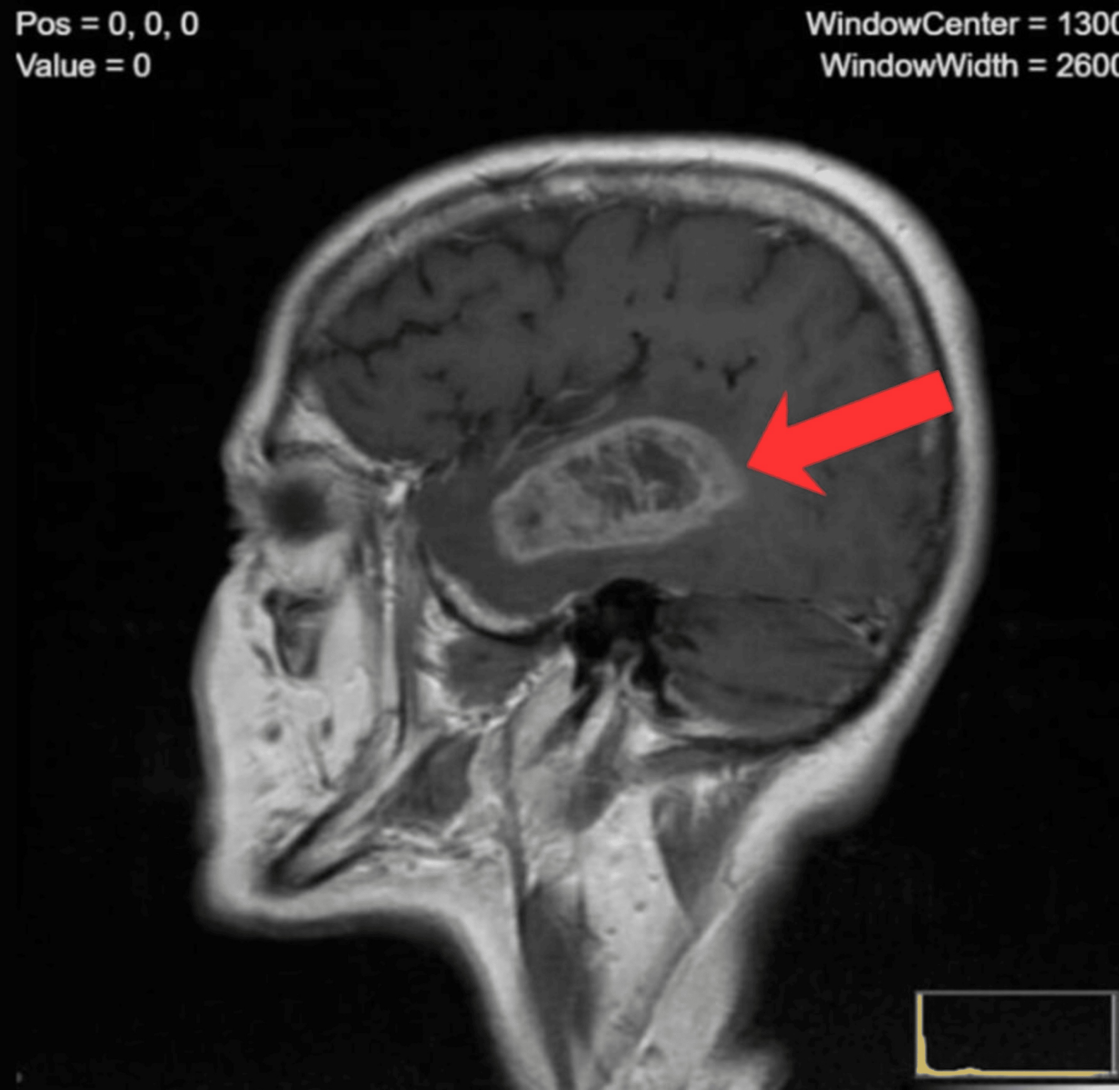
Sagittal contrast-enhanced MRI of a right temporal lobe mass Sagittal contrast-enhanced MRI demonstrating a large heterogeneous mass in the right temporal lobe with irregular ring enhancement and central necrosis. The lesion invades deep cerebral structures and produces a marked mass effect on the adjacent parenchyma. Surrounding vasogenic edema and compression of the right lateral ventricle are evident.
